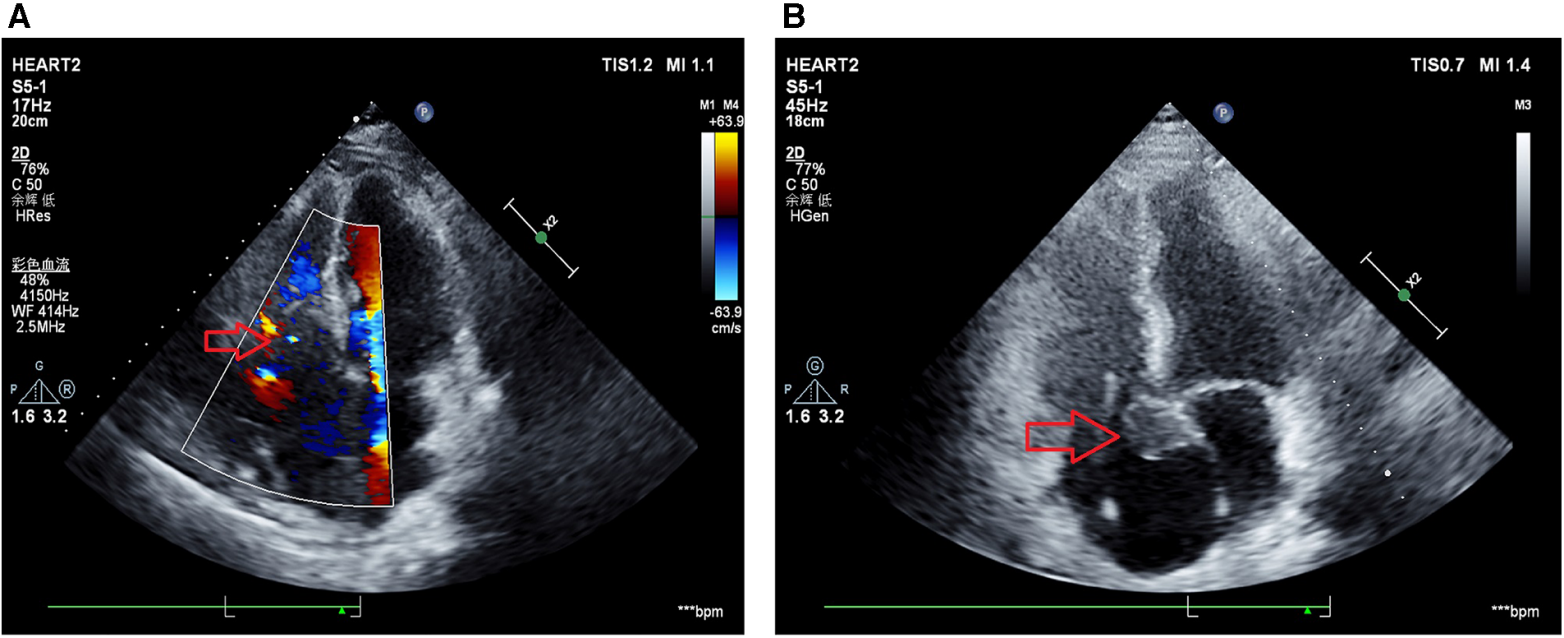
Echocardiography results after surgical resection. (A) Echocardiogram results at October 19, 2022 indicated a hypoechoic mass with irregular shape measuring approximately 85 mm × 54 mm is observed from the right atrium to the right ventricle. The boundary between the mass and the tricuspid valve is unclear, and the mass infiltrates the interatrial septum, suggesting tumor recurrence (with an increased size compared to pre-surgery) and severe tricuspid valve stenosis. (B) Echocardiogram results at November 1, 2022 indicated irregularly shaped solid mass in the right atrium, measuring 25 mm × 21 mm, adhering to the septal leaflet and inferior interatrial septum, with enlargement of the left and right atria, dilation of the aortic sinus, and severe tricuspid regurgitation.
